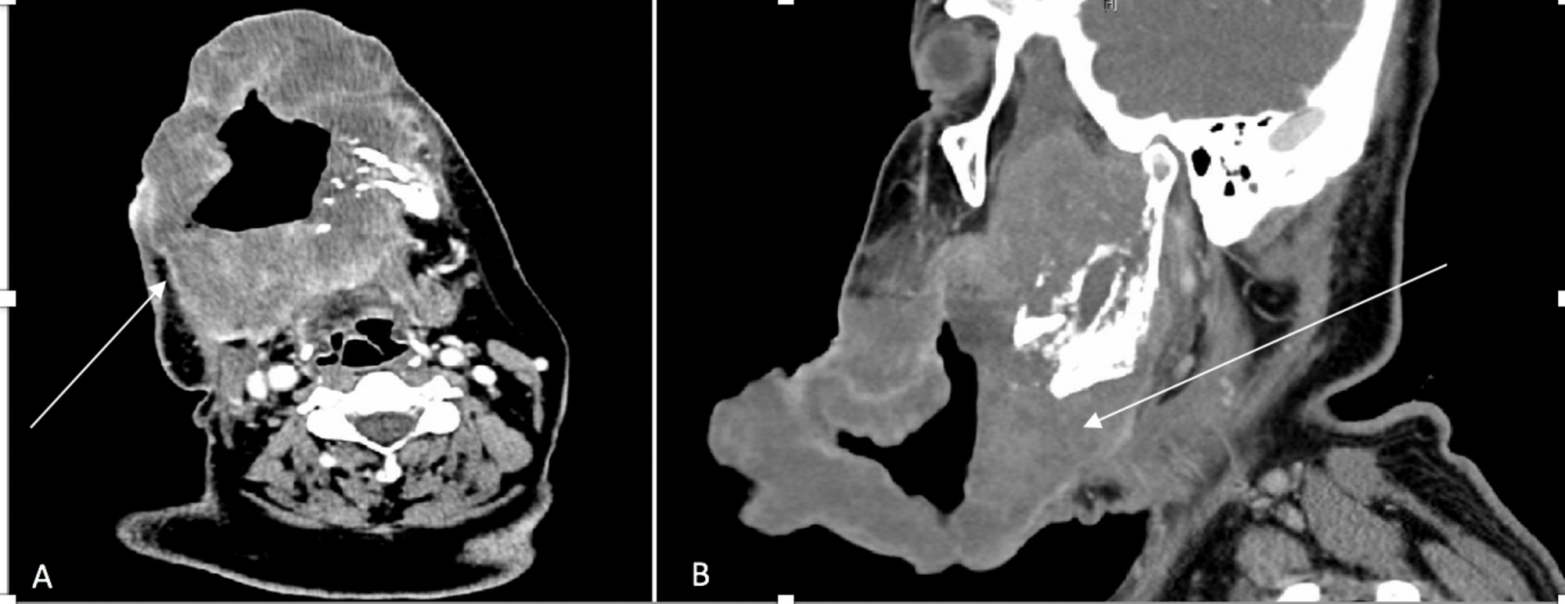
CT scan of neck with intravenous contrast CT scan of neck at presentation: (A) axial and (B) sagittal views. The white arrow points towards large infiltrative heterogeneously enhancing lesion extending from the floor of mouth to the right sided masticator space with associated erosion of the mandible and the right pterygoid plate.
